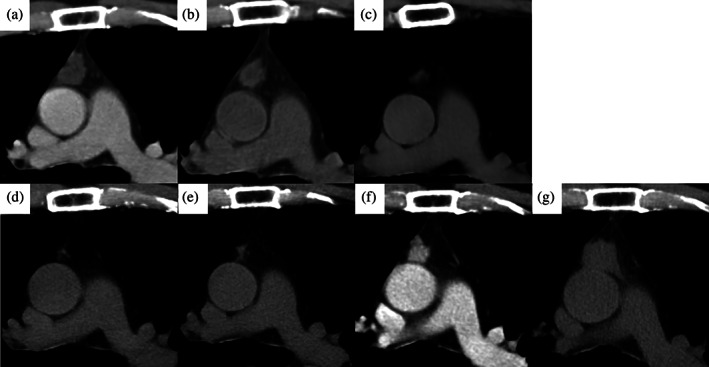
Anterior mediastinal computed tomography images of the mass. Images at (a) 3 years, (b) 4 years, and (c) 7 years after the mass was detected, showing that it had shrunk (d). Images at (e) 10 years, (f) 12 years, and (g) 13 years after the mass was detected, showing that it had grown.
